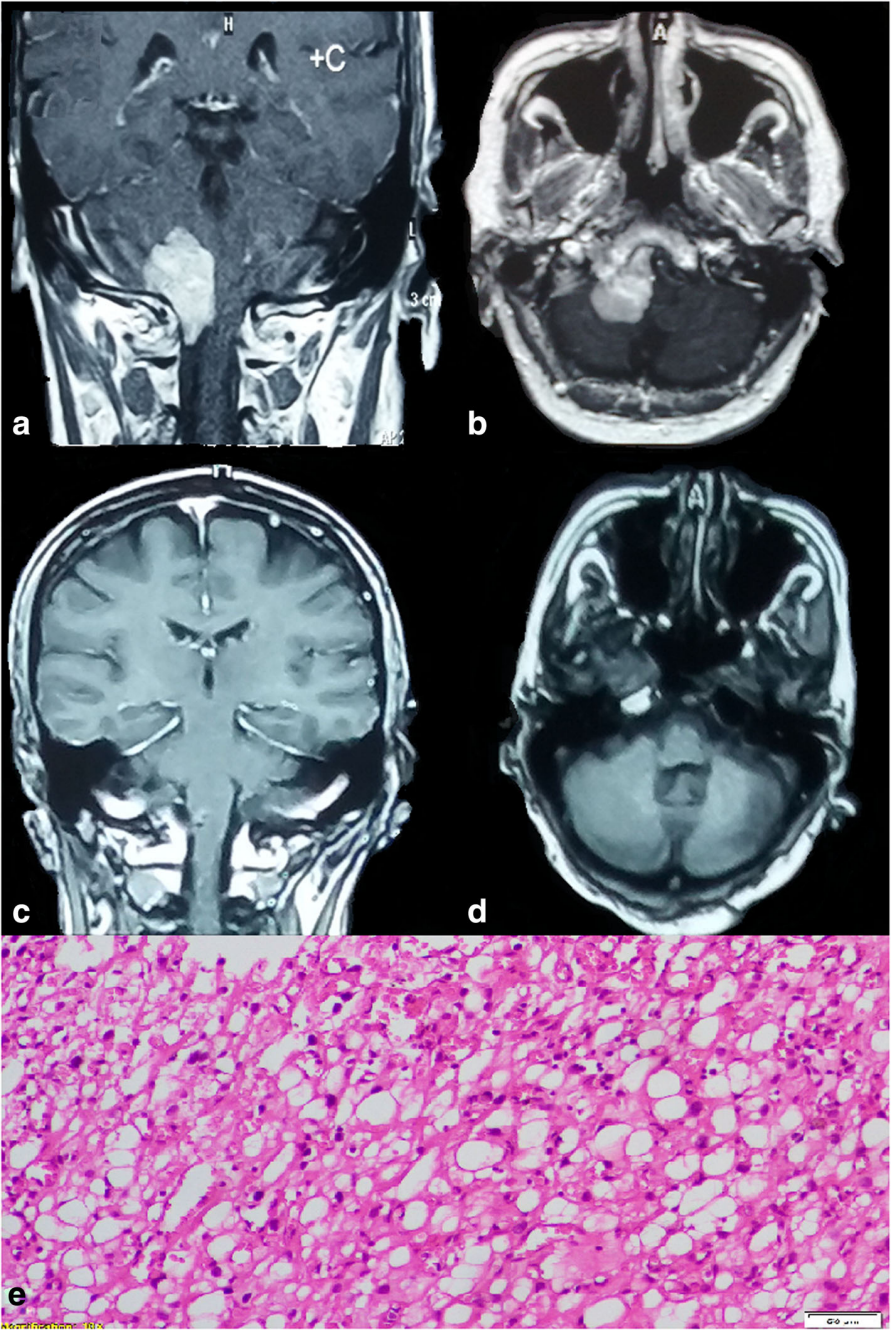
Foramen magnum meningioma. a Preoperative image, MRI with contrast (coronal) verified mass lesion compress the cervicomedullary junction and upper cervical cord. b MRI with contrast (axial) represented a ventral foramen magnum mass. c One year postoperative MRI with contrast (coronal) showed complete removal of the mass. d The same year MRI with contrast axial view demonstrated small ventral residual with complete medullary decompression. Geriatric Scoring System (GSS) score = 18. e The slide revealed a secretory variant of meningioma, WHO grade I
